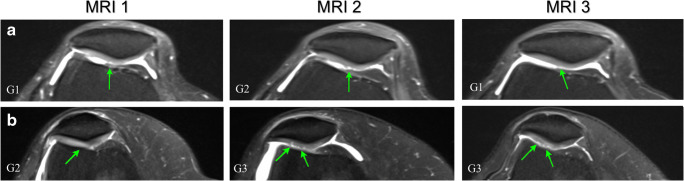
Axial proton-density fat-saturated MR images of two different knees with changes in the extent of chondral lesions of the patella: A) resolution at 6-month follow-up (MRI 3) of a lesion that previously developed from the pre-marathon scan to the 2 weeks post-marathon scan (MRI 1 to MRI 2), in the right knee of a 67-year-old woman; B) smaller lesion at MRI 3 in comparison to MRI 2. The extent of lesion falls within the same grade parameters; however, it is slightly smaller showing signs of reversibility, in the right knee of a 51-year-old woman. Cartilage abnormalities are indicated by arrows and the lesion grade (G) is included in the left bottom corner and is defined in the modified Noyes scoring system [17] (see Table 2)
